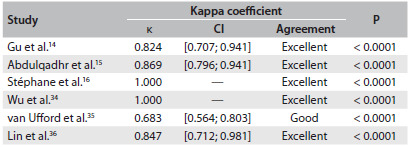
Agreement between WB-MRI and FDG-PET/CT for lymphoma staging in each of the studies included
